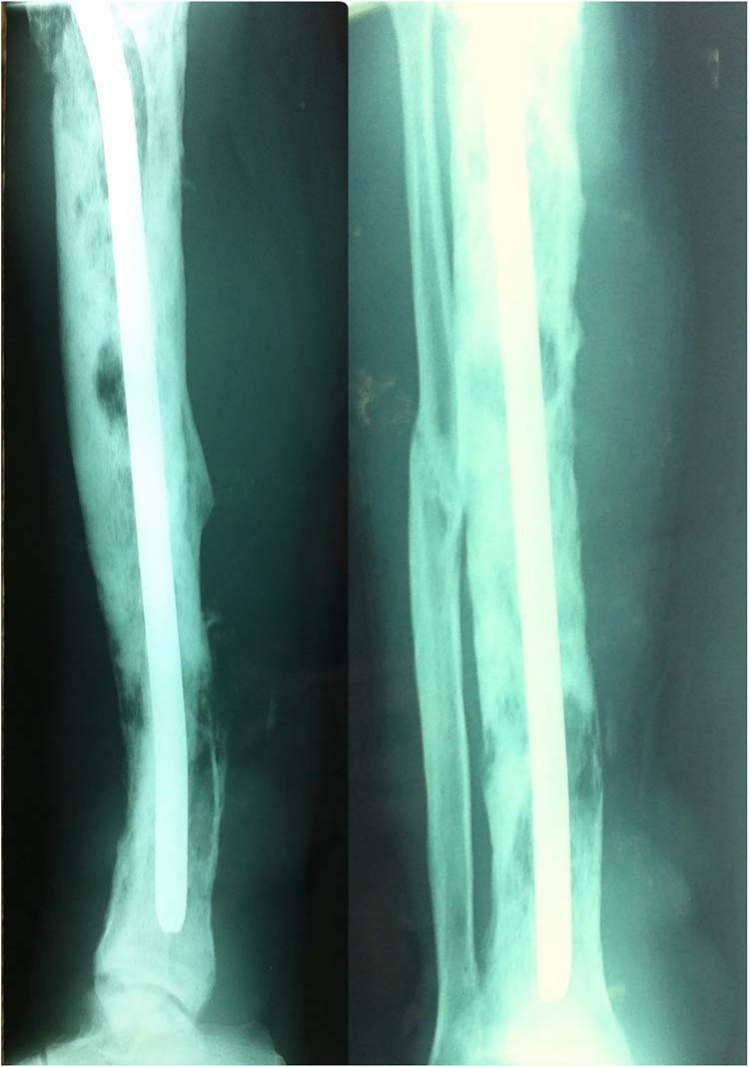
The X-ray showed a nail intramedullary in the left tibia, and multiple osteolytic lesions, heterogeneous, poorly limited with permeative destruction of the cortical bone.
